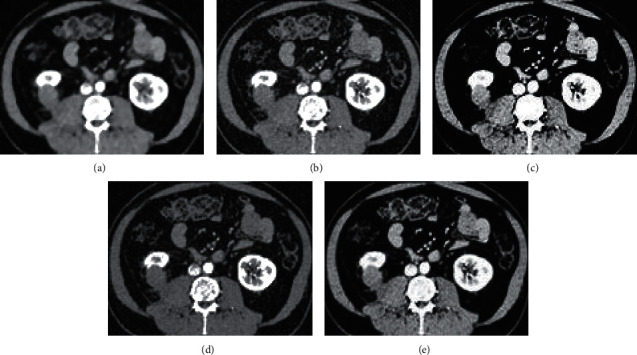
Comparison of image processing effects of DSST, WT, DNST, and O-DNST algorithms. (a) showed the image of CT plain scan; and (b–e) were images processed by DSST, WT, DNST, and O-DNST algorithms, respectively.
